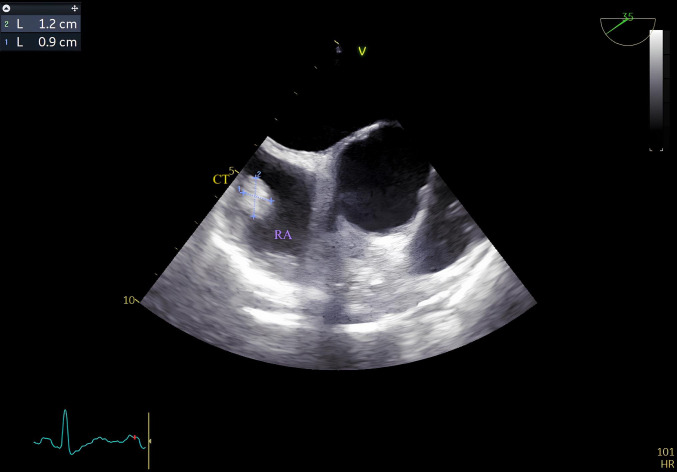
Transesophageal echocardiography (TEE) demonstrating a right atrial mass with dimensions of 12 × 9 mm. CT: crista terminalis, RA: right atrium.
